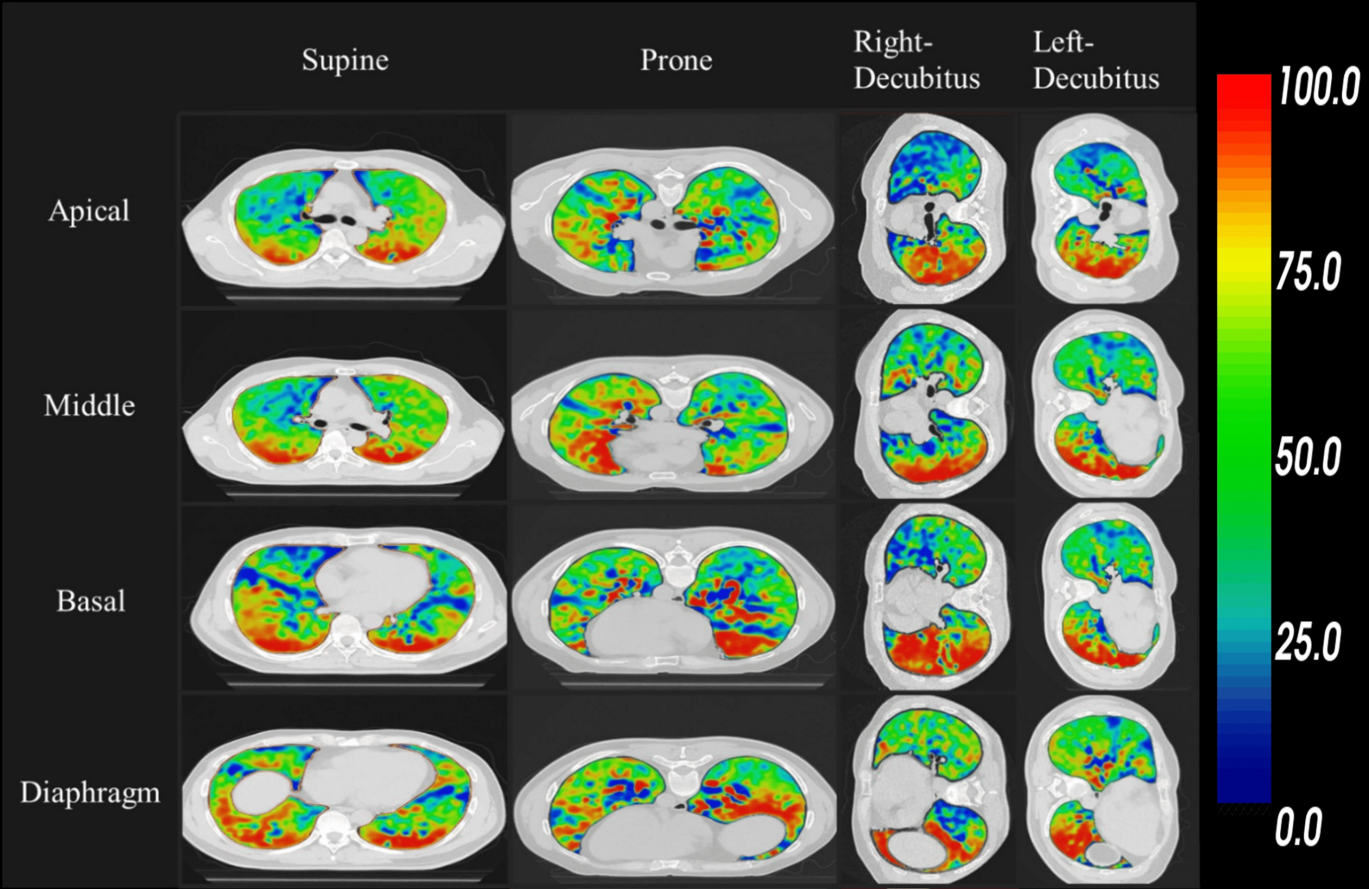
Comparison of lung ventilation images in each position by deformable image registration-based breath hold CT ventilation imaging (BH-CTVI) in the expiration and inspiration phase.
